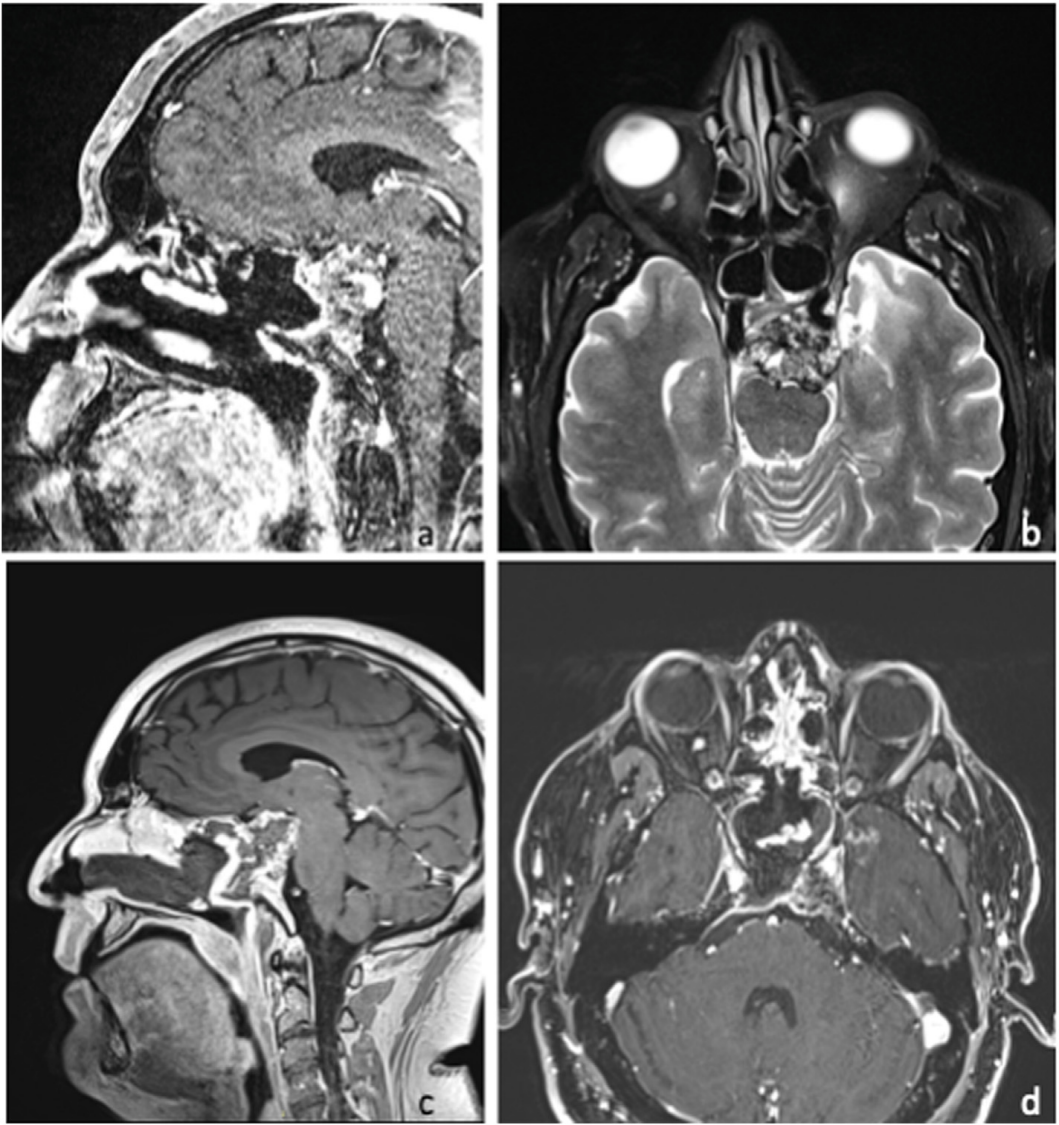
MRI, an exemplificative case of loco-regional recurrent chordoma, underwent surgery with a resective aim is reported. The patient of 32 years old was already treated by an EEA, followed by heavy particles radiotherapy. After 42 months, he presented with loco-regional recurrence, located in the middle/inferior third of the clivus with extra- and intra-dural extension, causing diplopia for CN VI palsy. A and B. T1-w with gadolium, sagittal and coronal view, showing the recurrent tumor. An EEA was planned with the aim to remove the tumor, followed by a second radiation therapy. C and D. T1-w with gadolium, sagittal and coronal view, demonstrating the complete tumor removal. Diplopia regressed and the patient is still alive at follow-up of 24 months, without any further tumor progression.
